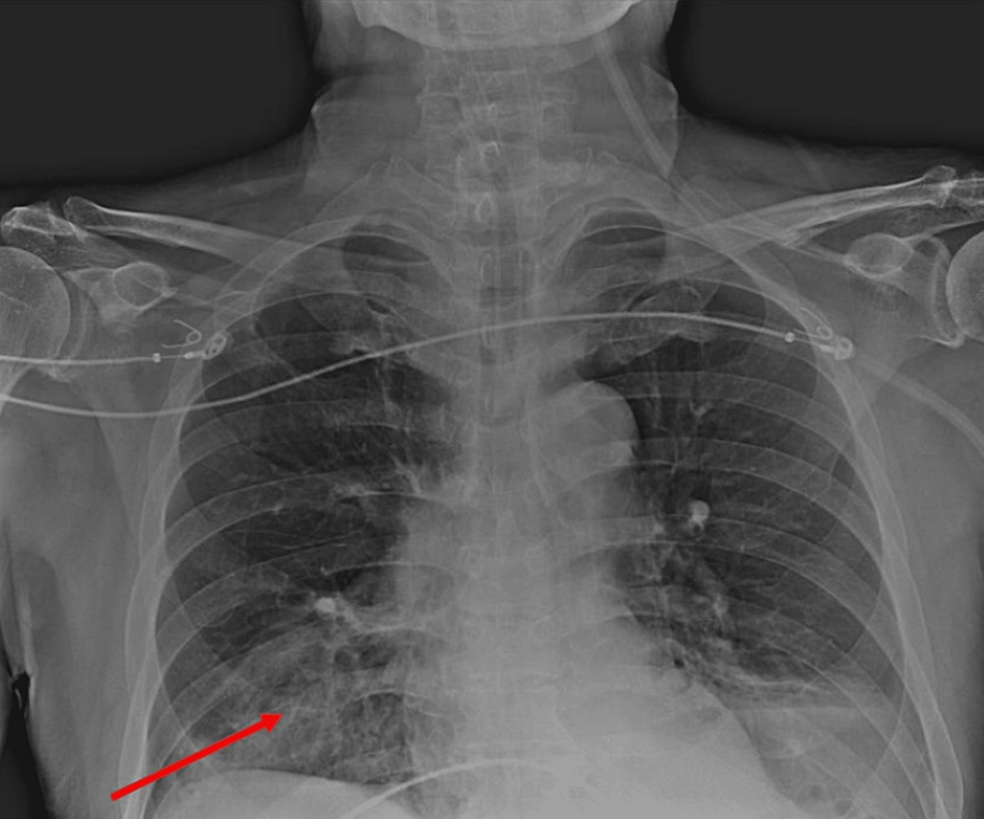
Chest X-ray of the patient. The patient has consolidations in the right lung on the chest X-ray.
